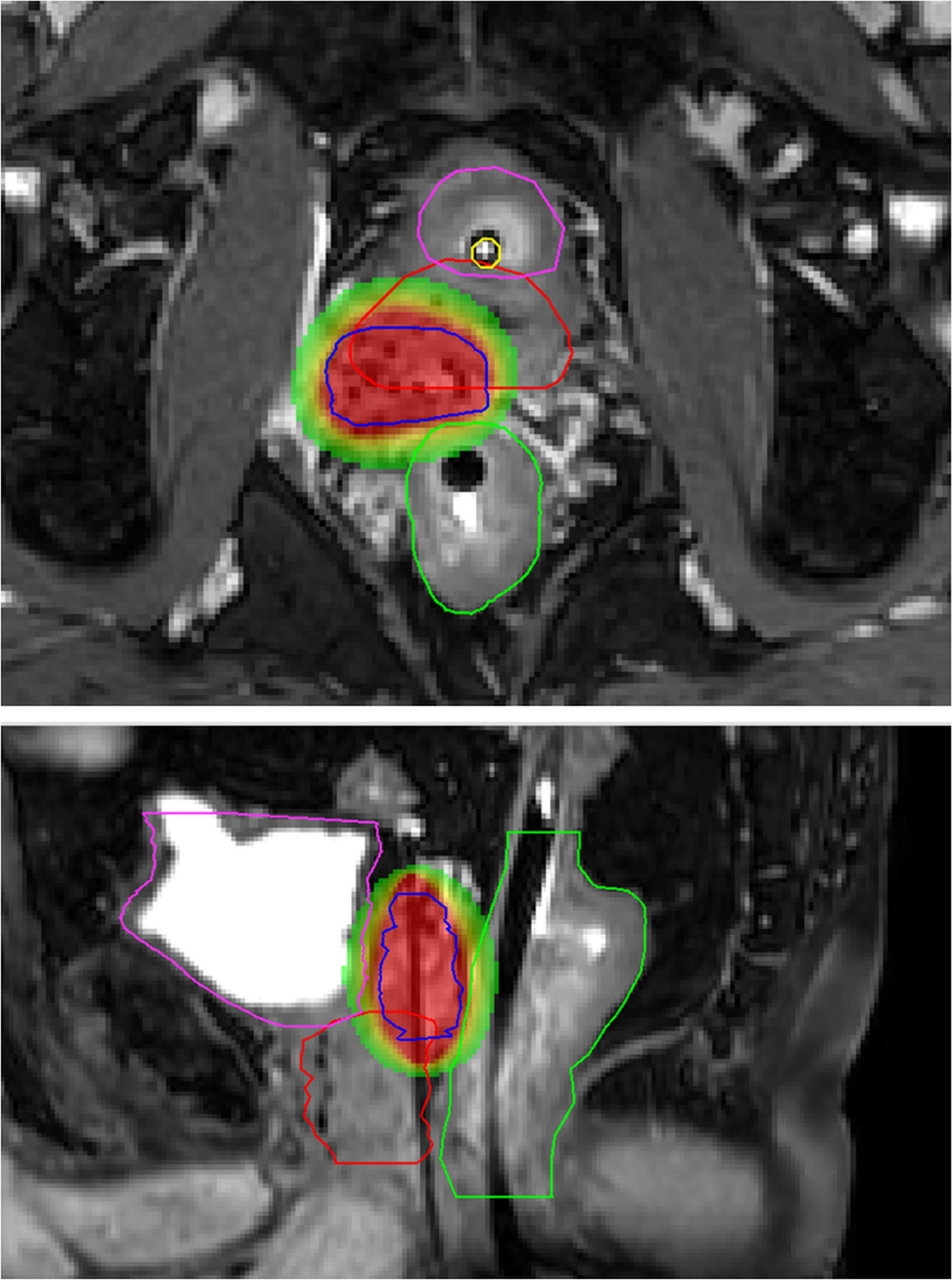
Dose distribution first MRI-guided focal salvage HDR-BT Transversal plane (upper magnetic resonance (MR) image) and sagittal plane (lower MR image) showing delineations of the bladder (purple), urethra (yellow), rectum (green), prostate (red), and gross tumor volume (GTV, blue) in the right dorsal peripheral zone and the right seminal vesicle. The images show signal voids from the catheters and previously implanted iodine 125 seeds. On the sagittal image, one of the catheters is visible within the GTV. In this case, the clinical target volume (CTV) was considered equal to the GTV because there was mainly seminal vesicle invasion. Radiation dose is displayed in colors, with red representing 19Gy and green representing 9.5Gy. MRI: magnetic resonance imaging; HDR-BT: high-dose-rate brachytherapy
